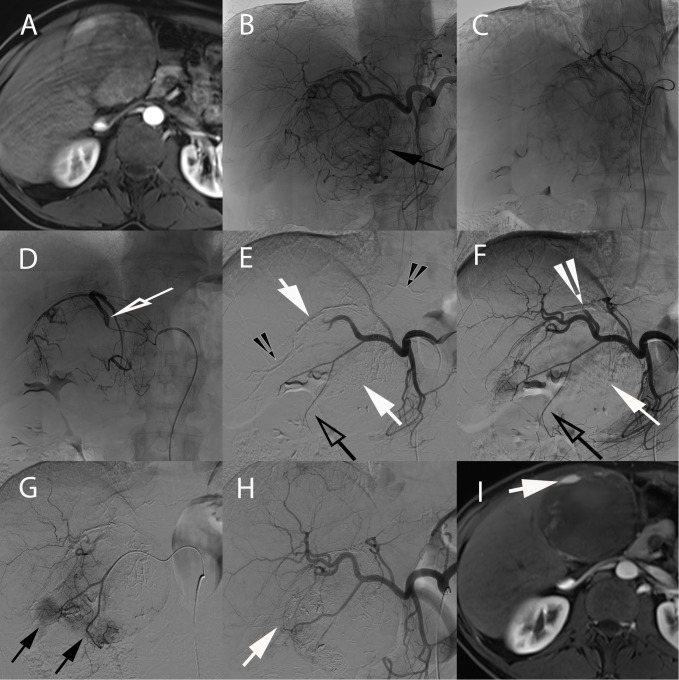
58-year-old male with hepatitis B and C cirrhosis and voluminous HCC of the left liver treated with radiopaque drug-eluting beads allowing real-time monitoring of treatment deposition. (A) Axial contrast-enhanced (arterial phase) T1-weighted MR image showing voluminous enhancing lesion of the left liver corresponding to an HCC. (B) Celiac angiography depicting large area of contrast blush corresponding to the tumor (black arrow). Superselective angiography with the microcatheter positioned in the left hepatic artery (C) and in the hypertrophied artery of segment IV (hollow arrow) (D), both supplying the tumor. Injection of radio-opaque drug eluting-beads (DC Bead LUMI loaded with doxorubicin) was performed from these 2 arteries. (E) Early and (F) delayed phases of same celiac angiography. (E) Delivered beads are readily visible, both within the tumor and peritumoral vessels (white arrows) and in non-target regions (black arrowheads). (F) The tumor blush is not visible anymore and complete blow stagnation is visible in segment IV artery (white arrowhead). However, no beads are seen at the bottom and lateral portion of the tumor [(E,F), black hollow arrows]. (G) Superselective microcatheterization and angiography from the cystic artery, showing areas of tumor blush consistent with residual untreated regions (black arrows). (H) Completion celiac angiography demonstrates treatment deposition in previously untreated tumor portion (arrow) and no residual tumor blush. (I) Axial contrast-enhanced (arterial phase) T1-weighted MR image performed 1-month after treatment showing large area of necrosis with minimal residual enhancing area in the anterior part of the tumor (arrow).
